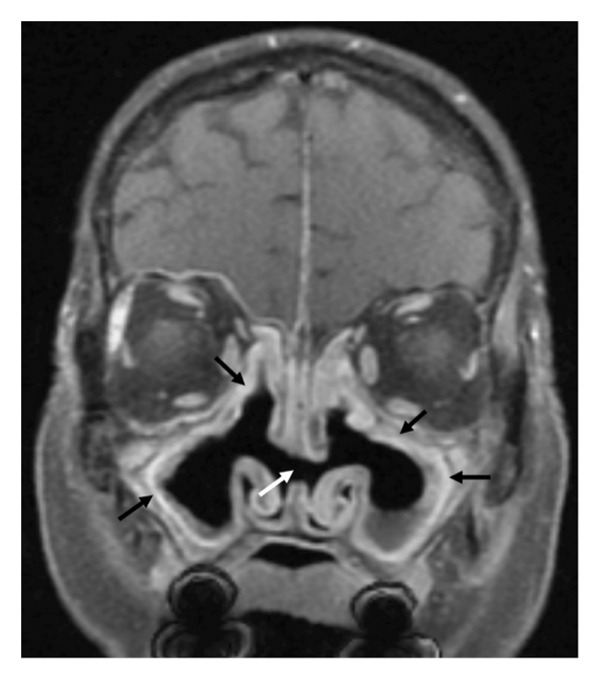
Magnetic resonance imaging of the head. Postoperative changes in the paranasal sinuses (black arrows) and nasal septal perforation (white arrow).
